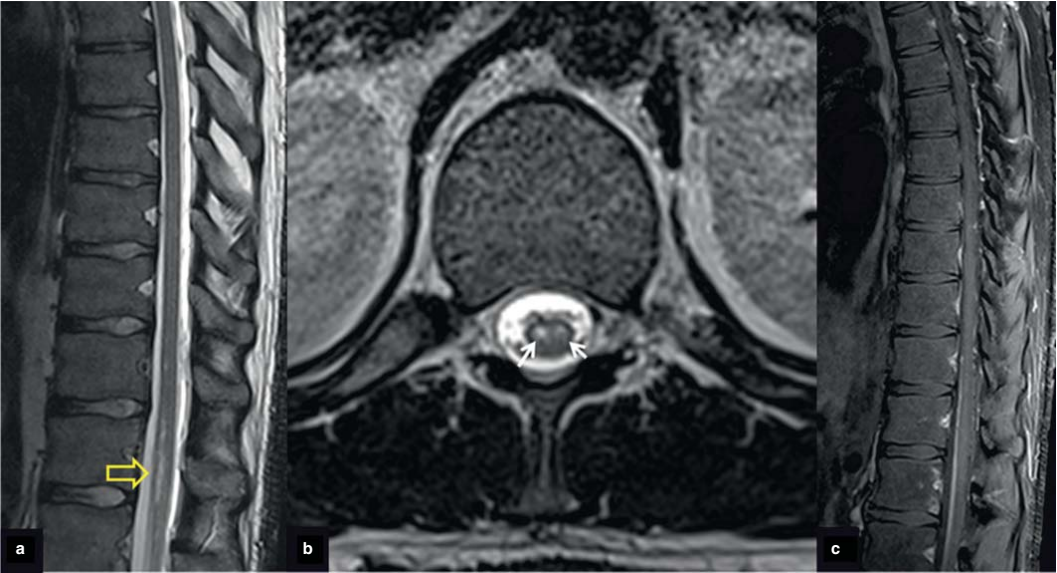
A follow-up MRI 6 weeks after the initial one shows well-defined borders of the lesion on the sagittal (a) and axial (b) *T*_2_ weighted MR images (arrows) and no enhancement on the fat-suppressed contrast-enhanced *T*_1_ weighted MR image (c).
